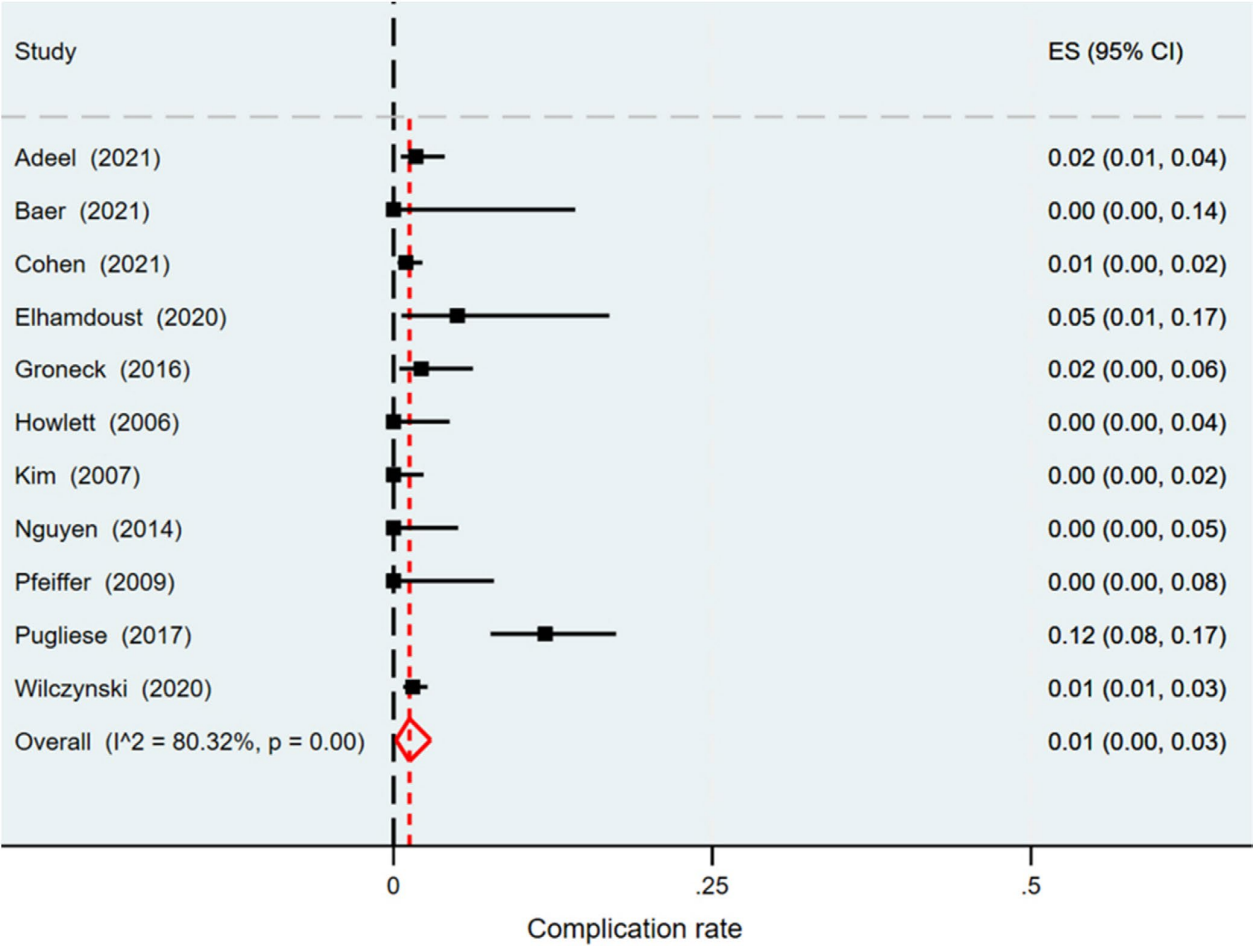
Forest plot of pooled complication rates of US‐guided CNB. Horizontal lines presents 95% CIs of each study. CNB, core needle biopsy; CI, confidence interval; US, ultrasound.
